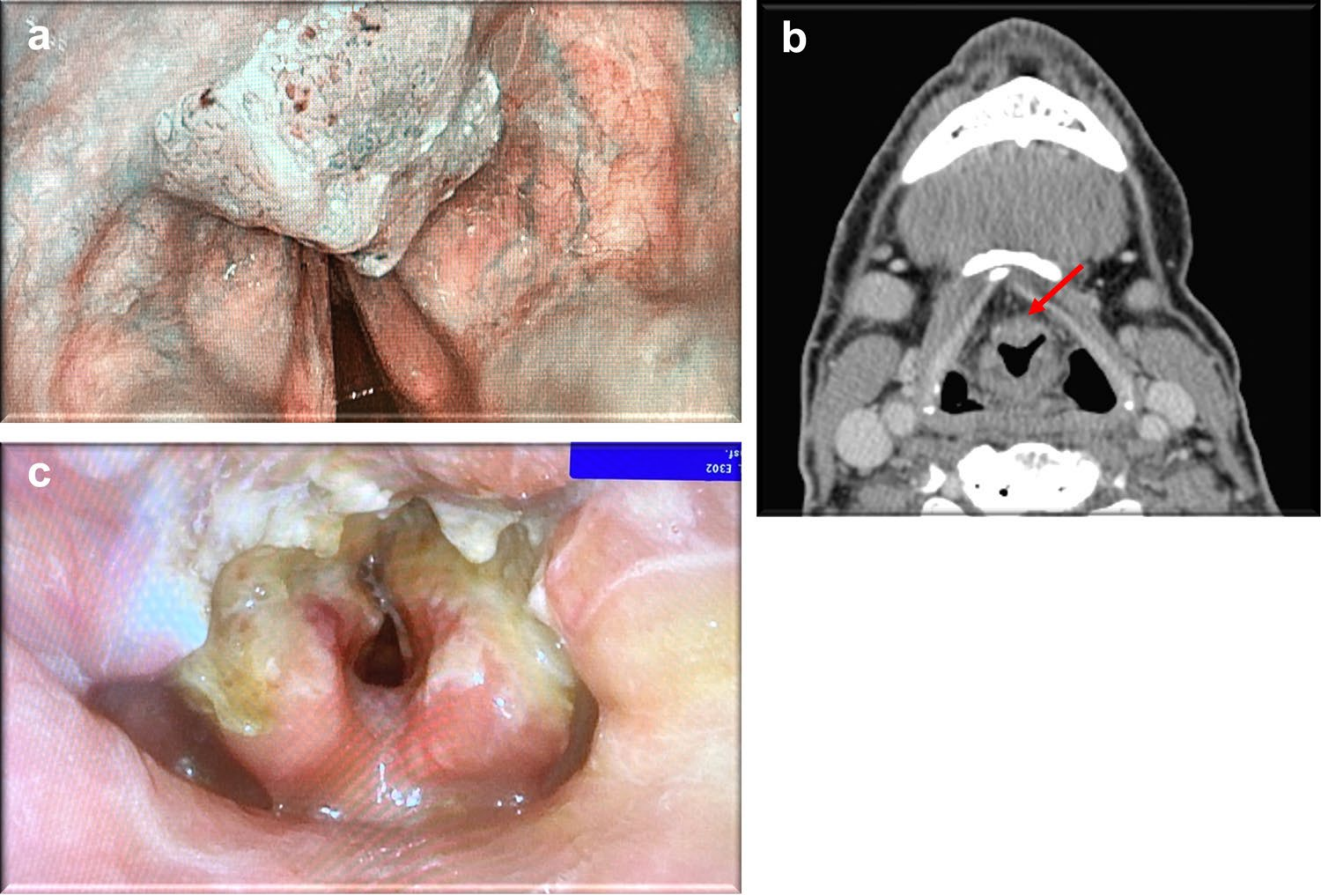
Case #2: Lesion of the laryngeal surface of epiglottis on narrow-band imaging (NBI) laryngoscopy (a) and axial computed tomography showing free pre epiglottic space (red arrow) (b), laryngoscopic image one day after surgery (c)
